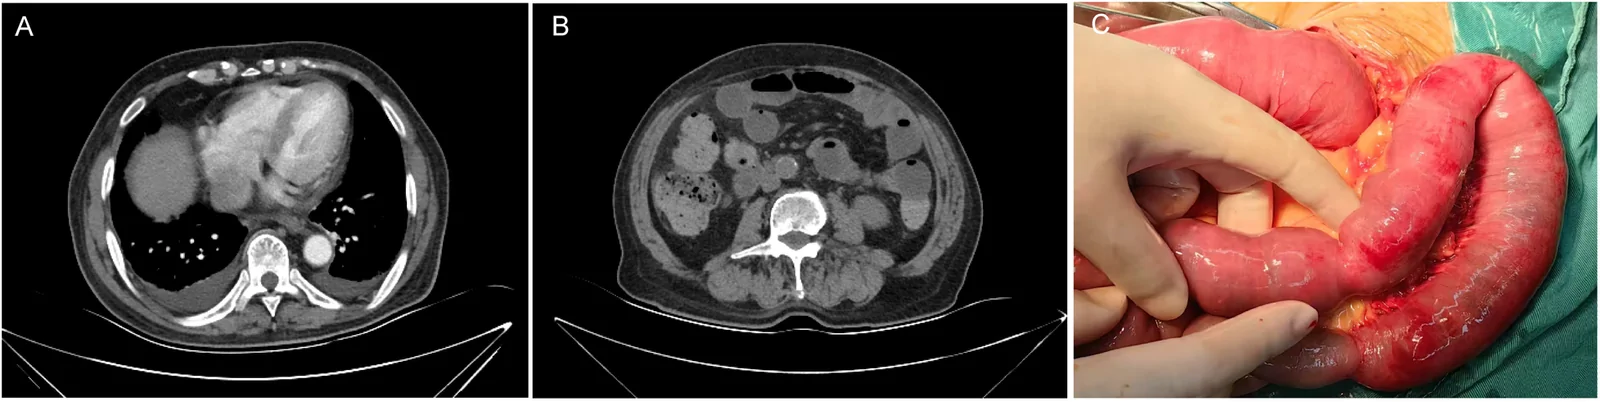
Imaging and intraoperative findings. (A) A chest CT on admission (9 February 2025): bilateral pleural effusions. (B) A whole-abdomen CT (18 February 2025): dilated small bowel with air–fluid levels, indicating mechanical small-bowel obstruction. (C) An intraoperative view obtained after adhesiolysis (25 February 2025), showing the previously herniated small-bowel segment with punctate hemorrhage, dusky discoloration, and mesenteric edema and congestion. No photograph was taken before adhesiolysis; panel C therefore documents bowel compromise and does not itself depict the omental adhesions or provide evidence of mycobacterial serosal fibrosis.
